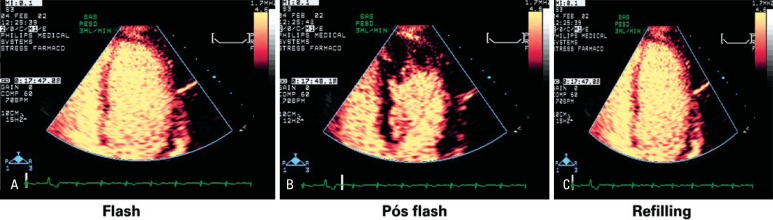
Echocardiographic images of real-time myocardial perfusion. (A) High ultrasound energy pulse (mechanical index - MI of 1.0). (B) Microbubble destruction in the myocardium. (C) Myocardial refilling by microbubbles
